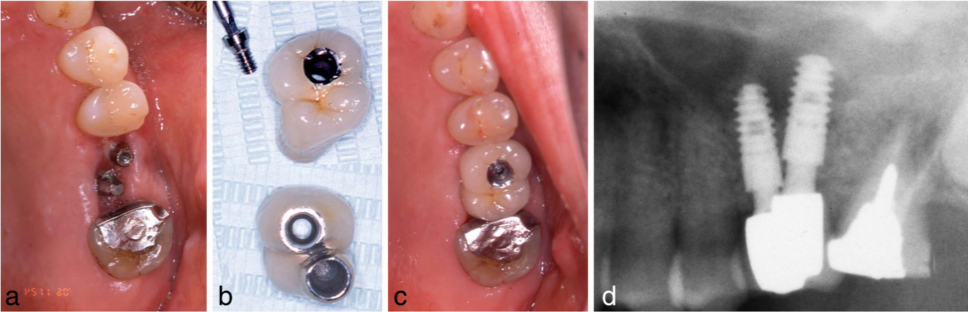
Case 1. November 2006. Six months after implant insertion. a–c The superstructure was attached with a screw. d Magnified panoramic X-ray film obtained immediately after superstructure placement
